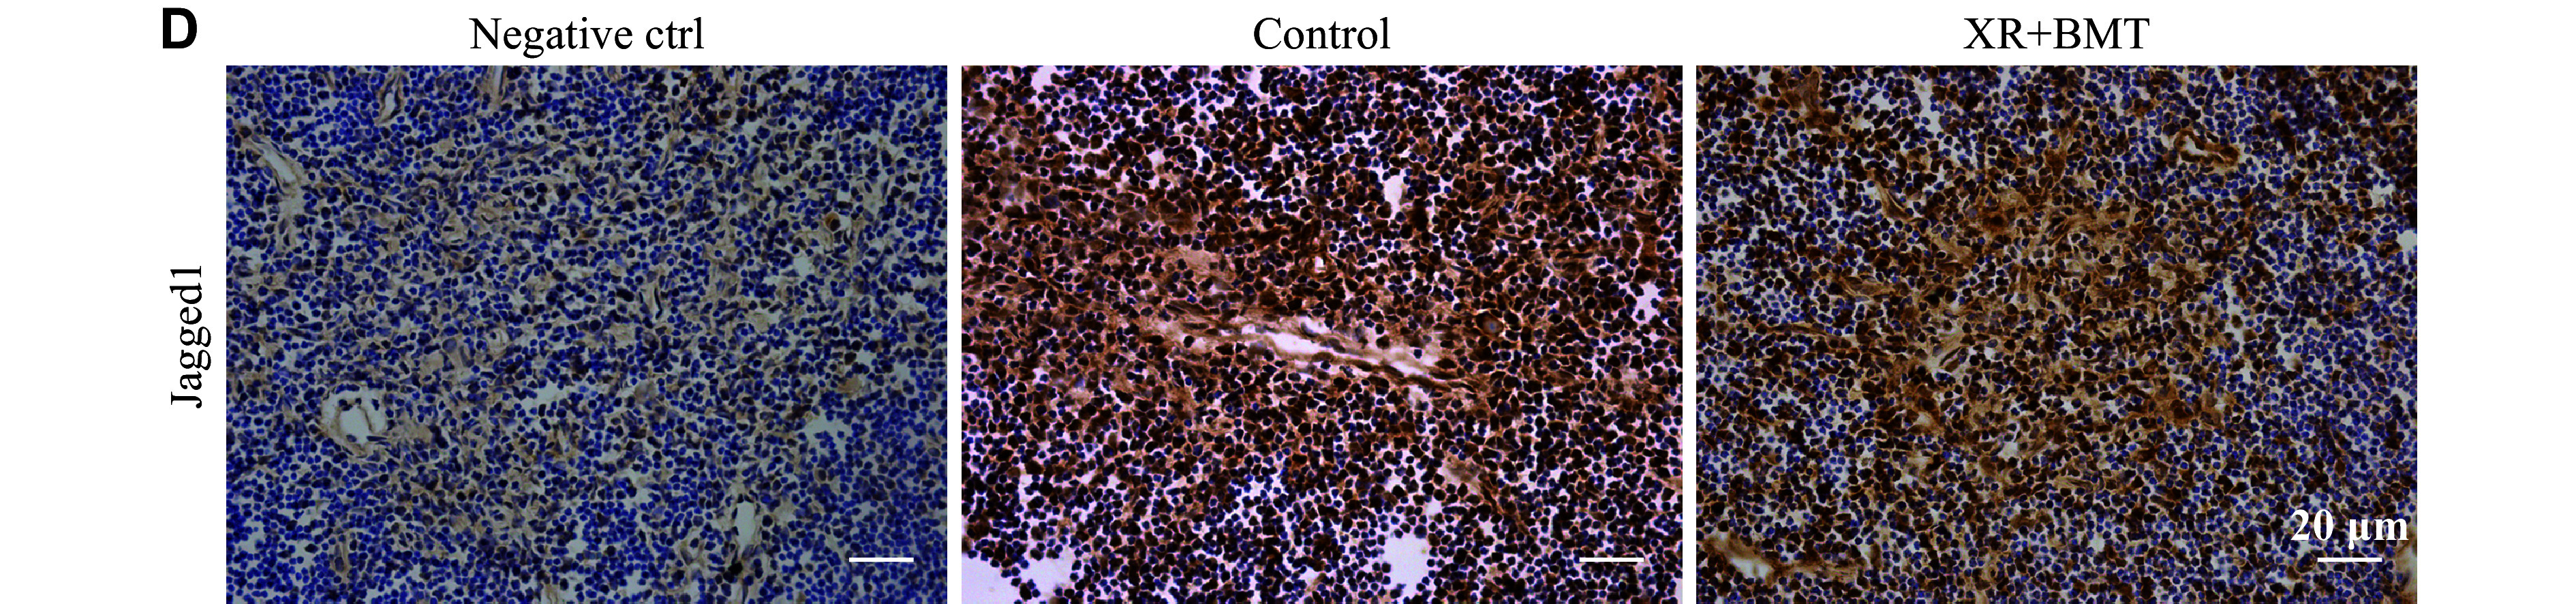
# Author Correction: X-ray irradiation selectively kills thymocytes of different stages and impairs the maturation of donor-derived CD4^+^CD8^+^ thymocytes in recipient thymus
